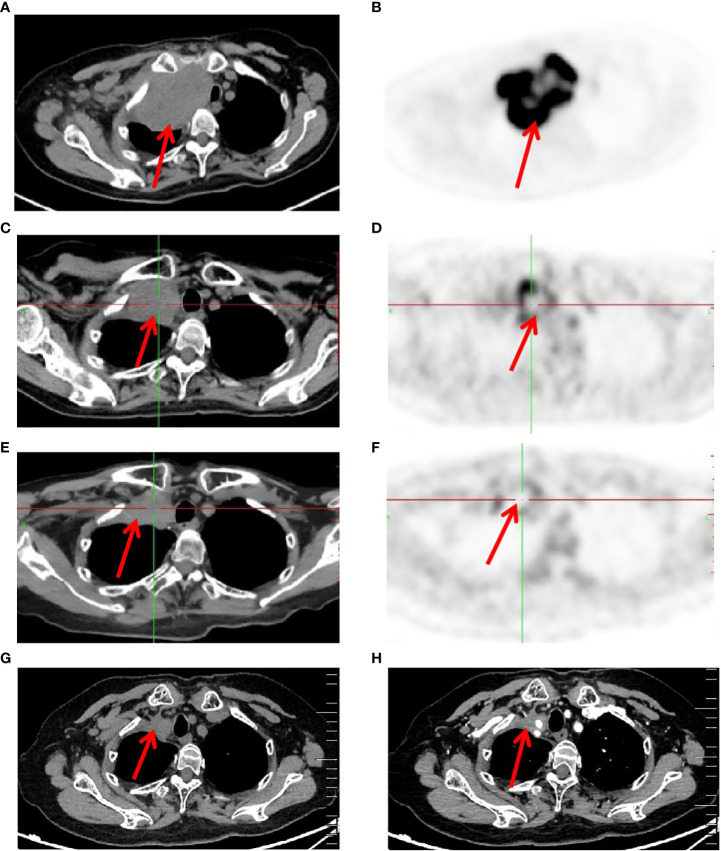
Imaging changes of patients before and after treatment. (A, B), Baseline PET-CT findings of the patient’s course (solid tumor, dmax = 70 mm * 44 mm, abnormal increase in FDG metabolism and SUVmax 28). (C, D), After 7 cycles of treatment (solid tumor, dmax = 54 mm * 43 mm, abnormal increase in FDG metabolism and SUVmax 7.4). (E, F), After 15 cycles of treatment (solid tumor, dmax = 46mm * 33mm, and no tumor activity and SUVmax 2.4). (G, H), After 34 cycles of treatment Computer Tomography results (solid tumor, dmax = 22mm * 20mm).
